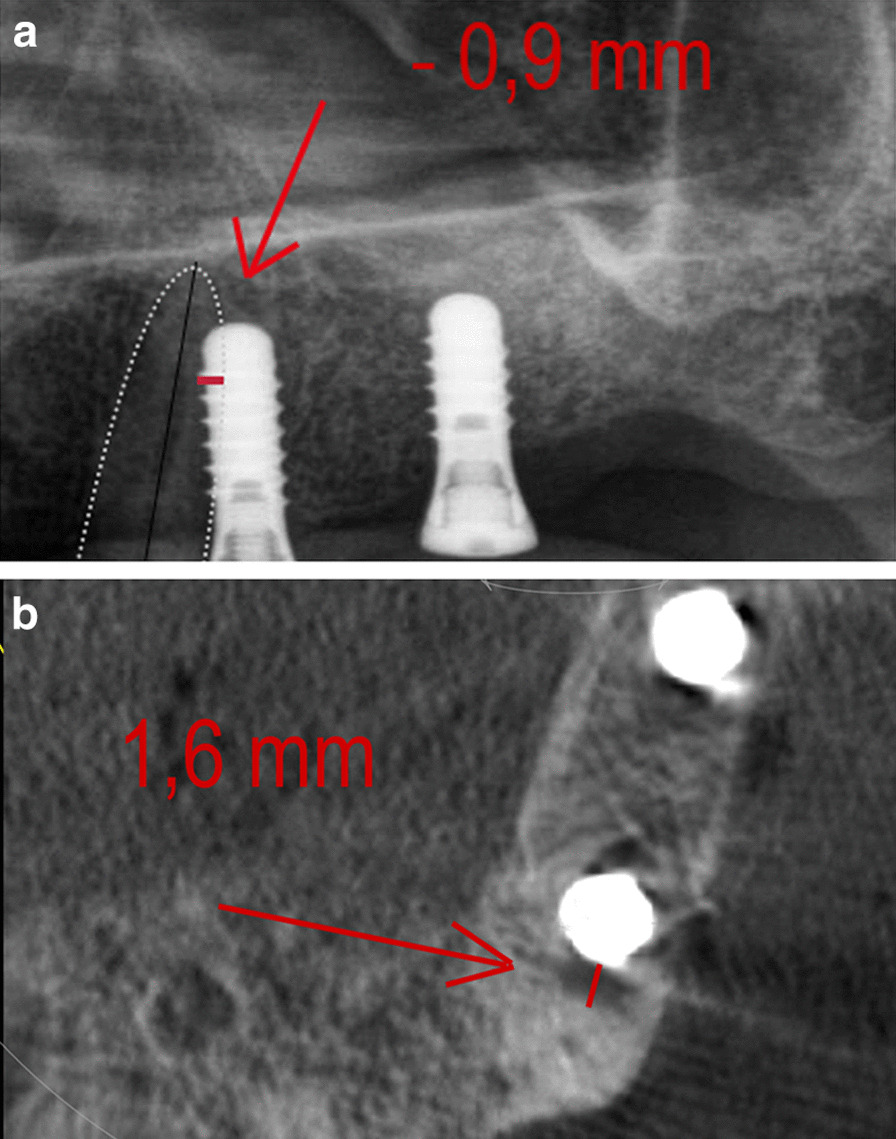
IRD measuring technique on PAN (a) and CBCT (b) with overlapping structures in PAN. a Outer surfaces of implant and neighboring root (dotted white line) are assessed on PAN images. At the area of smallest distance, i.e. greatest overlapping distance, between implant and adjacent root surface the local implant thread was used as reference point for IRD measurement (red line). b Outer surfaces of neighboring implant and root were assessed at the level of the reference thread on axial CBCT images. IRD represents distance between implant and adjacent root surface at the level of the reference thread (red line)
